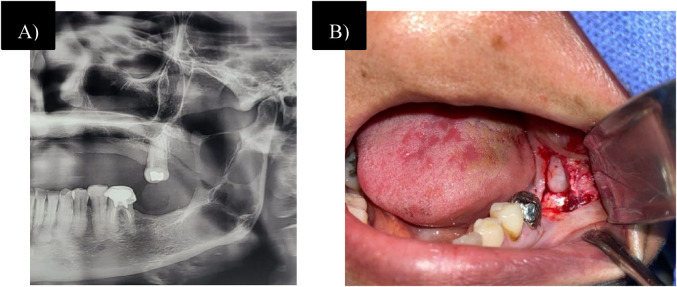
Clinical images. (A) Radiograph showing a multilocular lytic lesion. (B) A soft, whitish lesion was observed.
